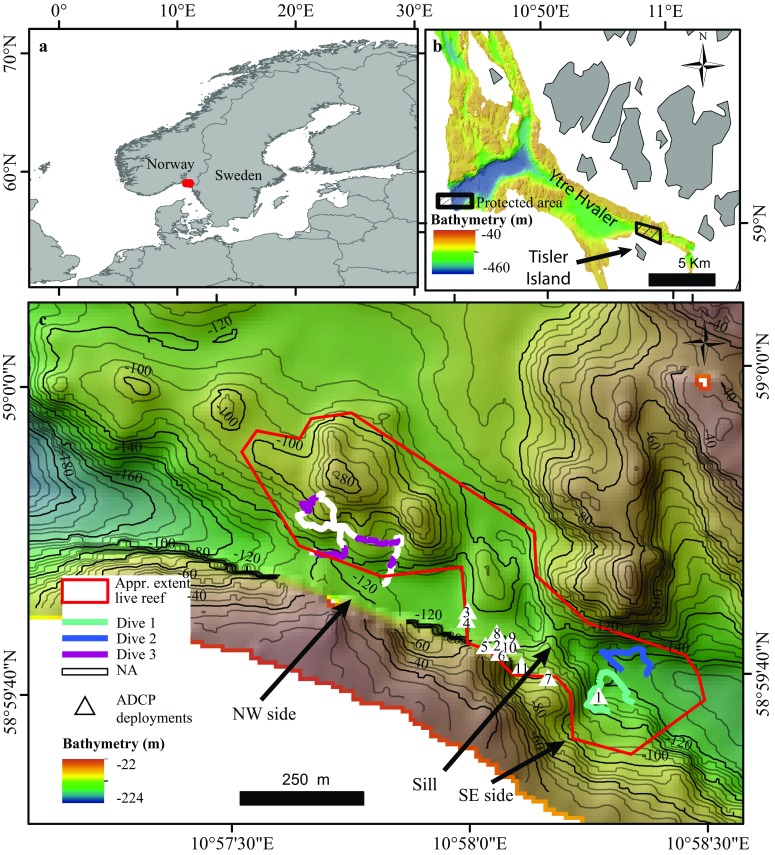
a Location of Tisler Reef in Norway. b Location of protected area surrounding Tisler Reef (black box) in Kosterfjord. c Locations of remotely operated vehicle dives. The white part of dive 3 represents data which was not available (NA) for analysis. The white triangles with numbers show locations of the acoustic Doppler current profiler (ADCP) deployments
